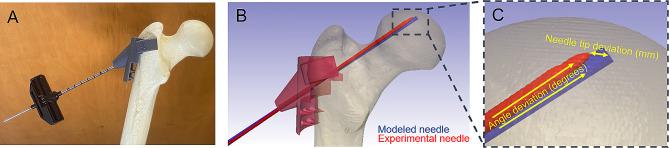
(A) 3D-printed guide fitted to a foam cortical shell femur is displayed. The femur was modeled using a dual-energy CT scan and the custom device was fitted using 3D modeling software. A Jamshidi needle, utilized subsequently for an AVN decompression device, was positioned using the modeled femur. A second dual-energy CT-scan was obtained after drilling the foam cortical shell femur with the Jamshidi needle. (B and C) The position of the Jamshidi needle after drilling was compared to the 3D-modeled position
